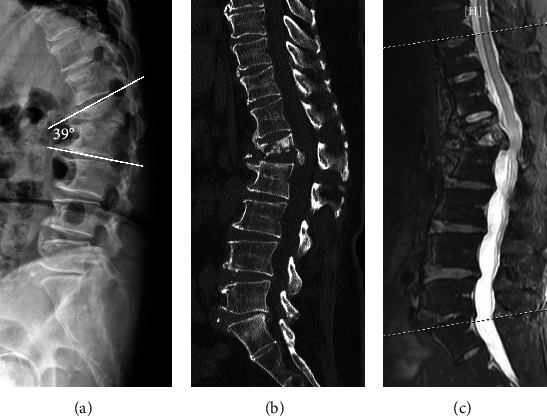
(a) Lateral radiographs showed a collapsed nonhealed L1 fracture and local kyphosis (39 degrees). Sagittal CT (b) and MRI (c) show a retropulsed bone fragment which compromised the spinal canal.
